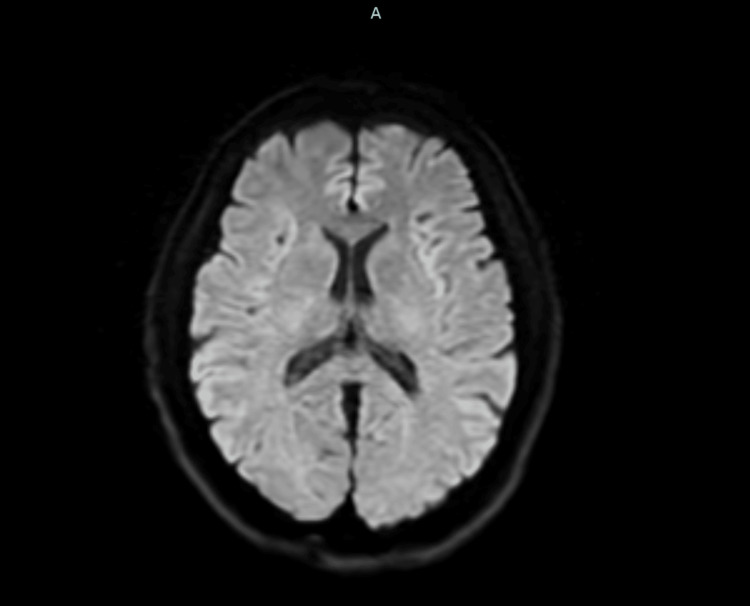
Axial diffusion-weighted MRI of the brain at the level of the basal ganglia demonstrating no diffusion restriction
